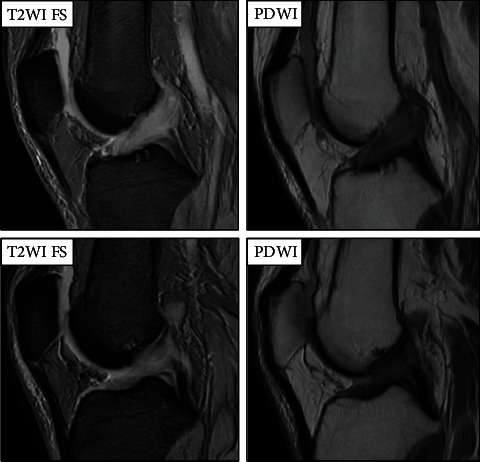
MRI showed diffuse thickening of both ACLs, which are called celery stalk sign, with high inhomogeneous intensity in the T2-weighted and proton density weighted images.
